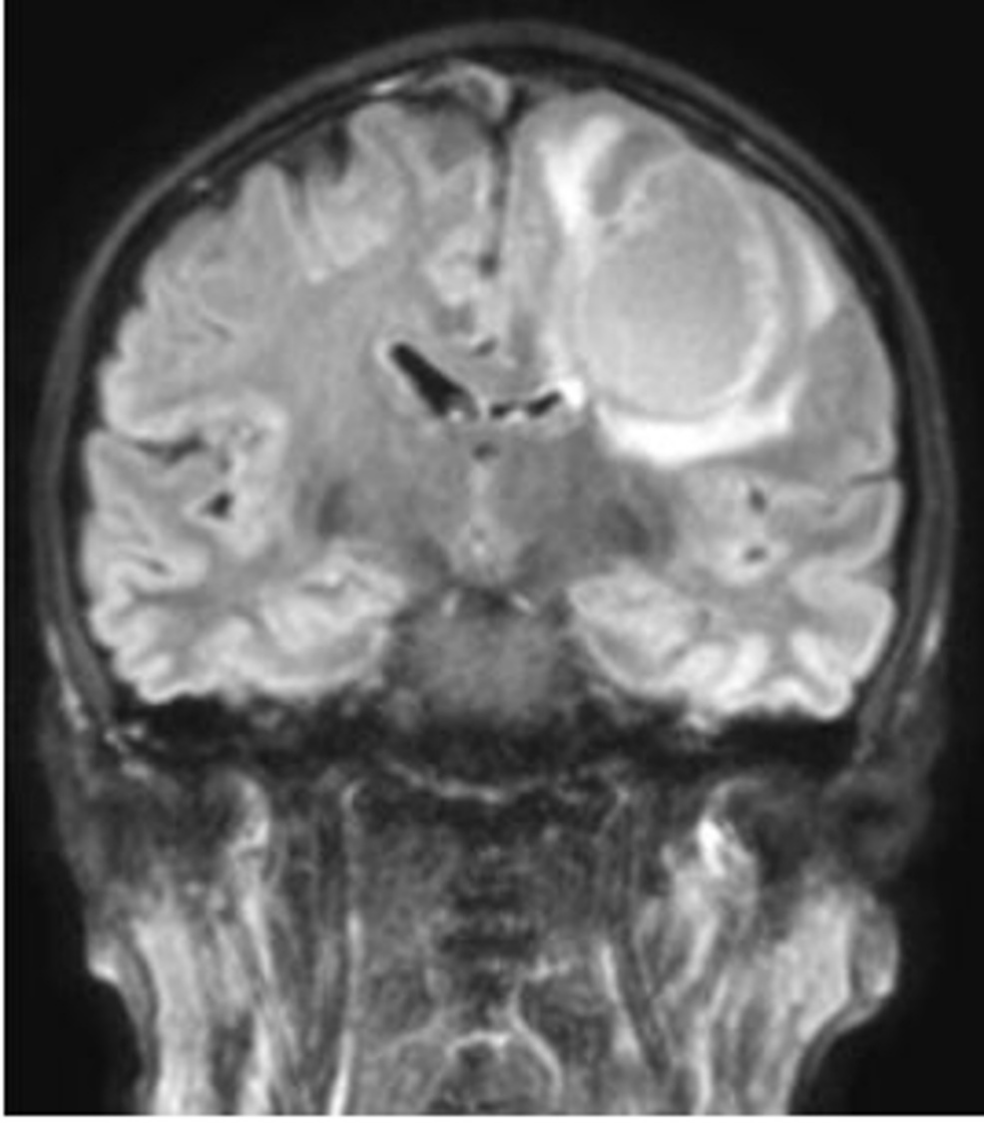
Lesion is partially suppressed on coronal fluid-attenuated inversion recovery (FLAIR).
